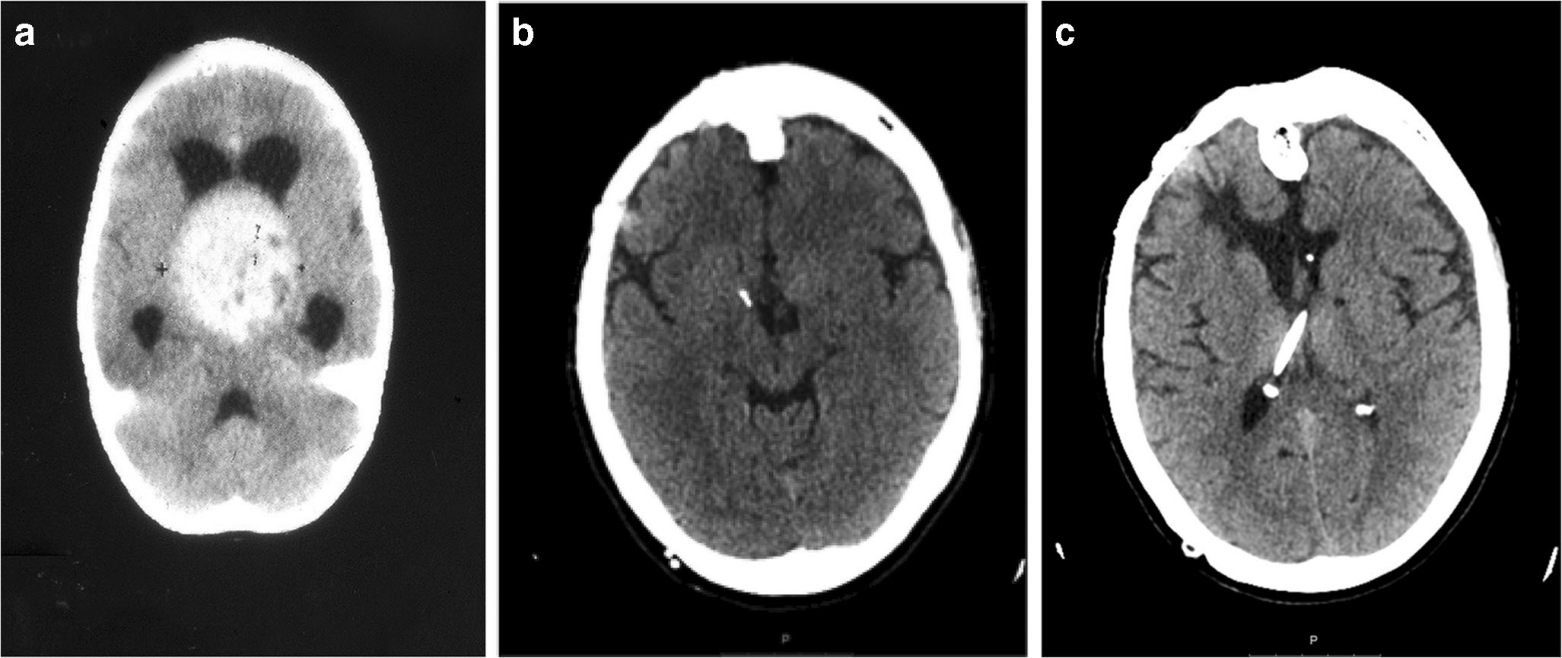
A 2-year-old girl presenting in 1981 with severe headache, ataxia, and visual impairment. CT demonstrated a large 3rd ventricular tumor (a). After STR of low-grade astrocytoma and subsequent hormonal substitution, she has also been shunt-dependent for HC and needed repeated resections in 1994 and 2009 due to progression of residual tumor. In spite of left-sided amaurosis, she is today at the age of 40 years in part-time work with a CT indicating tumor control (2019, b, c)
